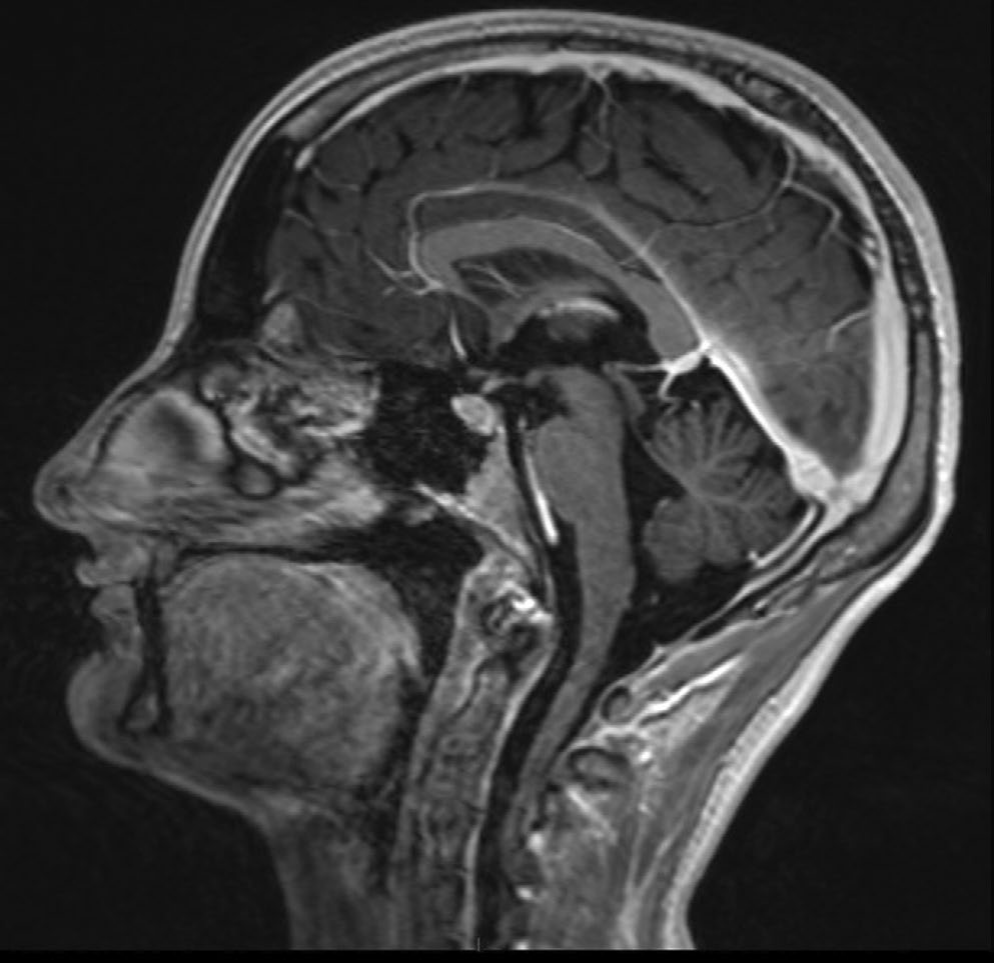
FIGURE 1 Preoperative Brain MRI
